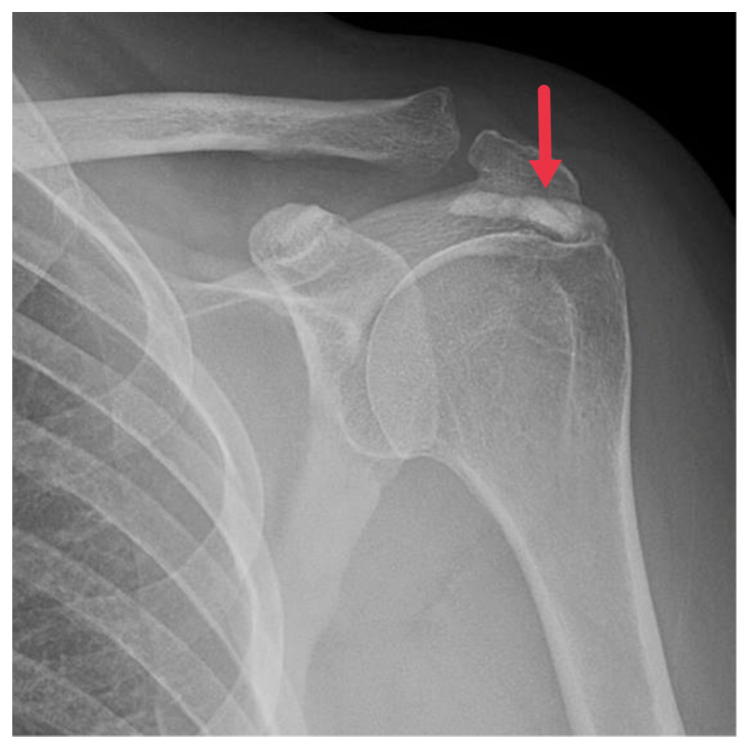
Frontal radiograph of the left shoulder demonstrating a well-defined calcification in the supraspinatus tendon (red arrow).
